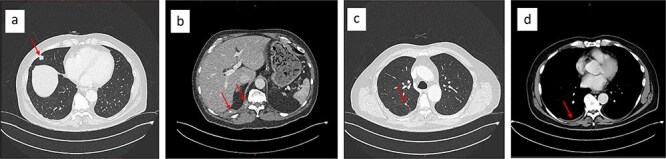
Chest CT showing partially calcified stable 15 × 13 mm subpleural lesion in the lateral middle lobe (a), a paravertebral mass at the level of the T11/12 vertebral foramen, and an intercostal lesion at the level of T10–12 (b). Nodular changes along the oblique fissure (c), and a mild right-sided pleural effusion (d).
